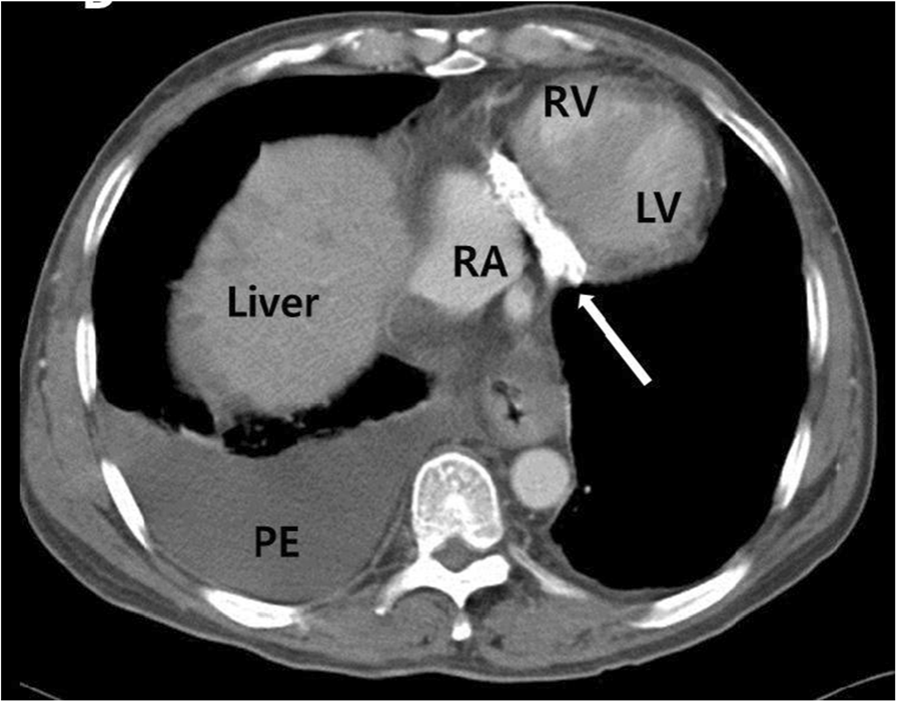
A chest CT revealing dense calcification (arrow) along atrioventricular groove, and pleural effusion (PE).
